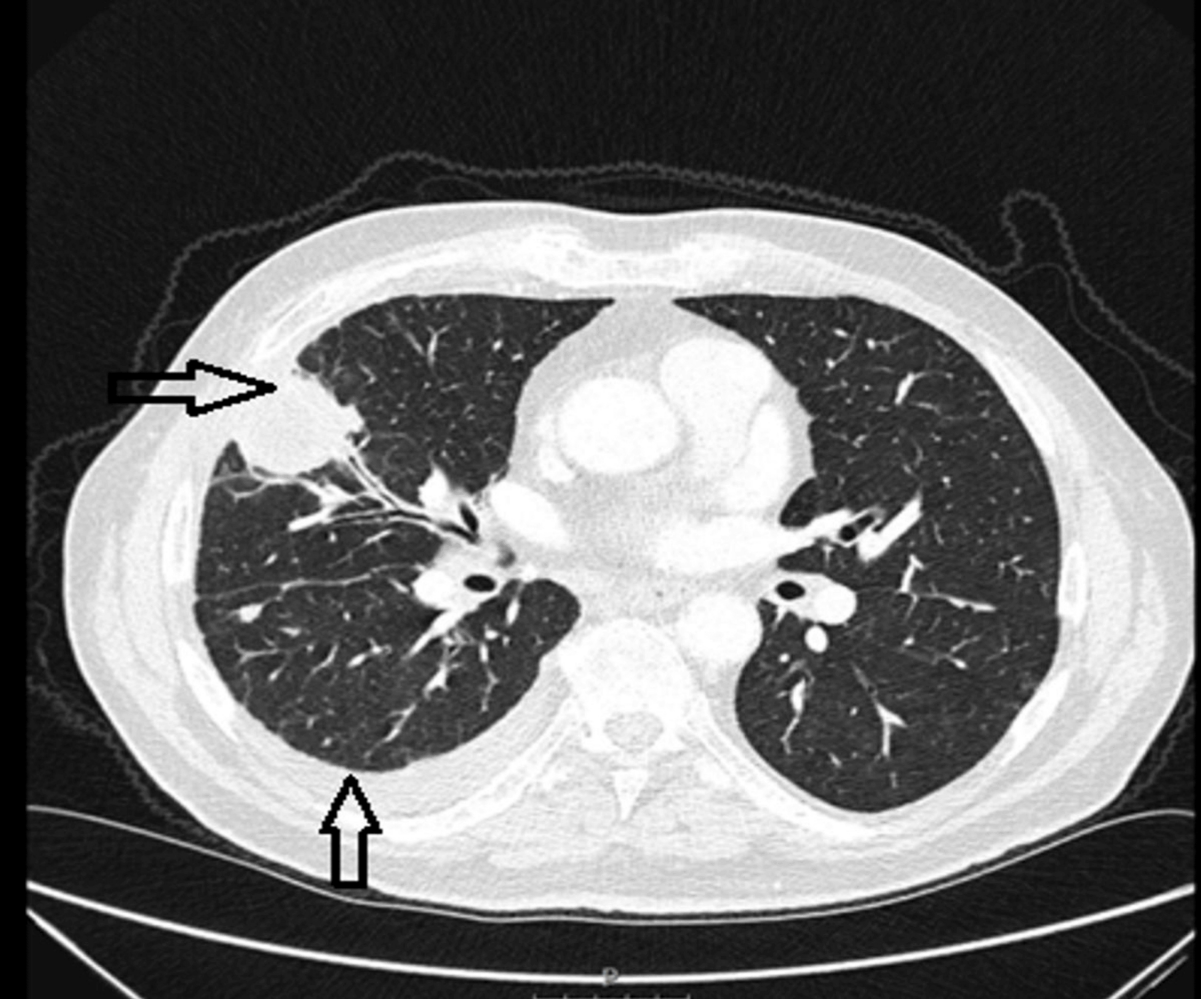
The CT scan of the chest's lung window showed a pleural-based, low-density mass in the periphery of the middle lobe, measuring 38 × 36 mm, and small right-sided pleural effusion.
